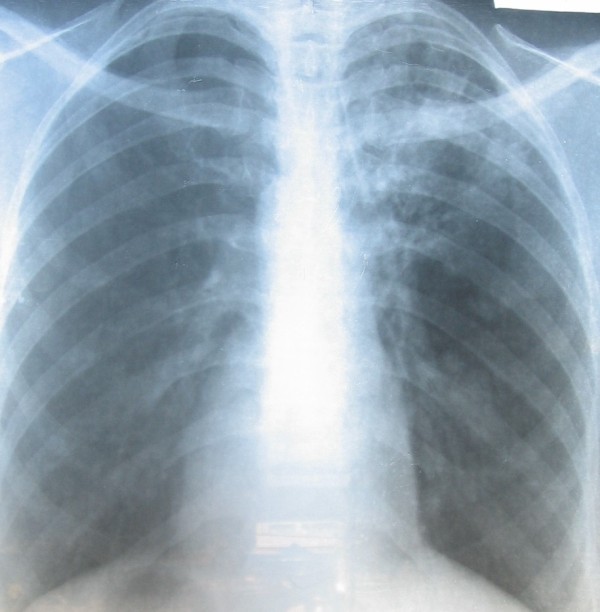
Chest radiograph of our patient on presentation to our clinic.
